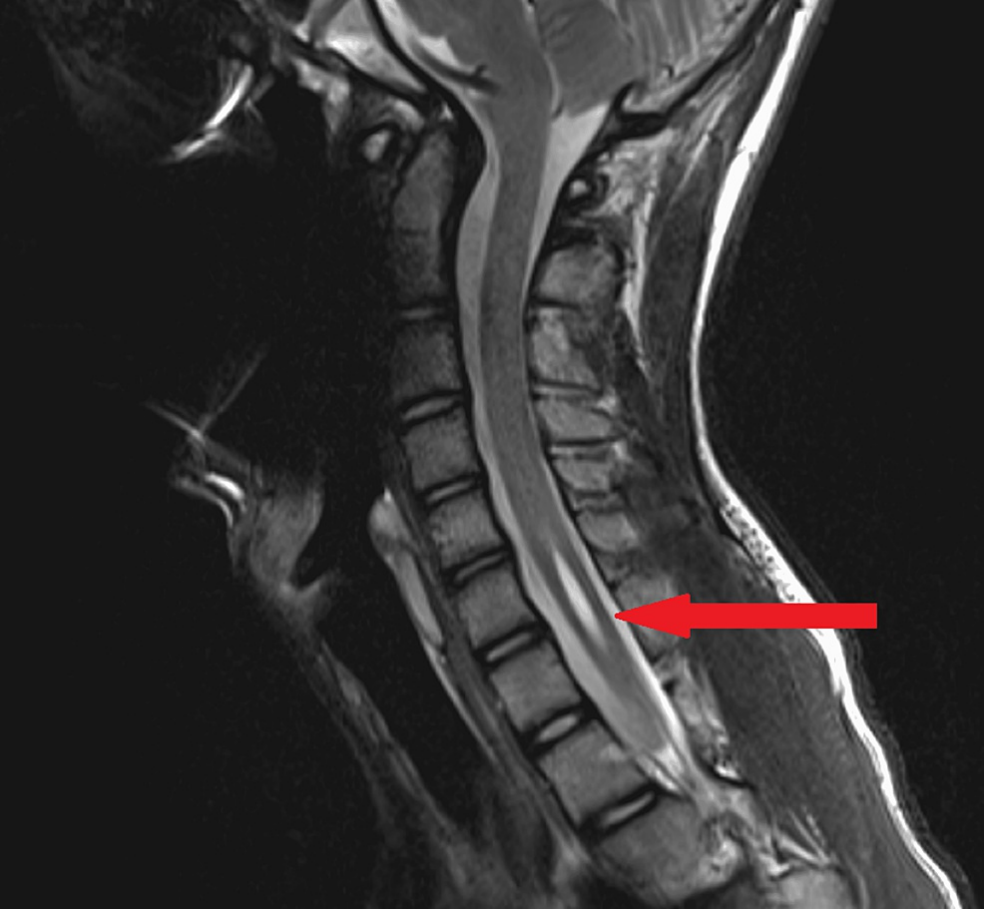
Pre-operative MRI spine. Note the short segment syringomyelia. It extends from the level of the C5/6 disc space to the inferior end-plate of C7, superior to the level of the ciliospinal centre of Budge and Waller.
